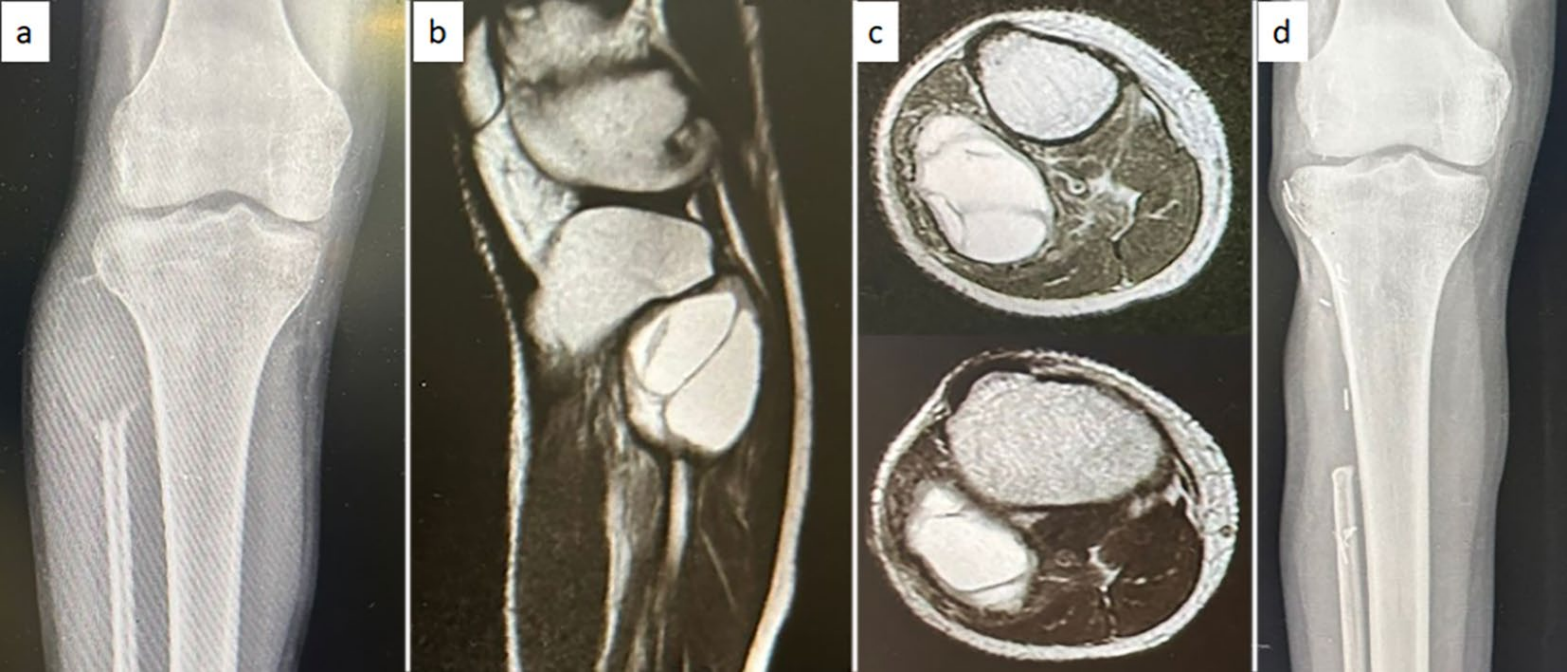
Radiological images of proximal fibula GCT; a pre operative radiograph showing expansile lytic lesion, b & c MRI images in sagittal and axial plane showing secondary ABC (Aneurysmal bone cyst) component, d post operative radiograph after proximal fibulectomy
